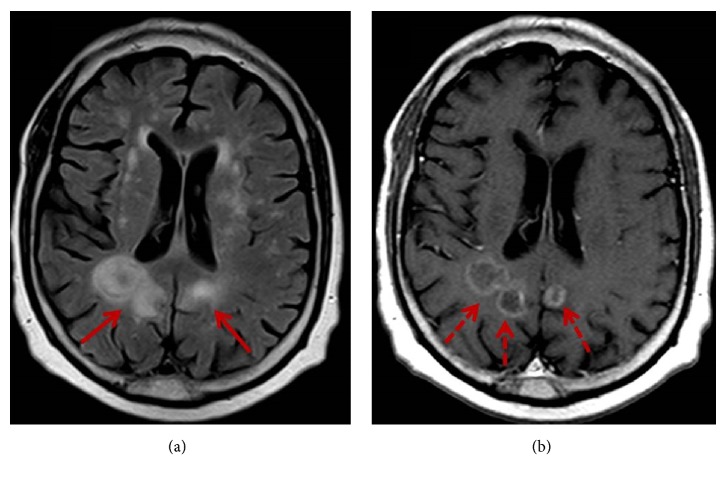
Axial T2-weighted FLAIR (a) and T1-weighted postcontrast MR images (b) demonstrate ring enhancing lesions in the bilateral parietal lobes (dashed arrows) at the level of the basal ganglia with surrounding vasogenic edema (solid arrows) in keeping with brain metastases.
